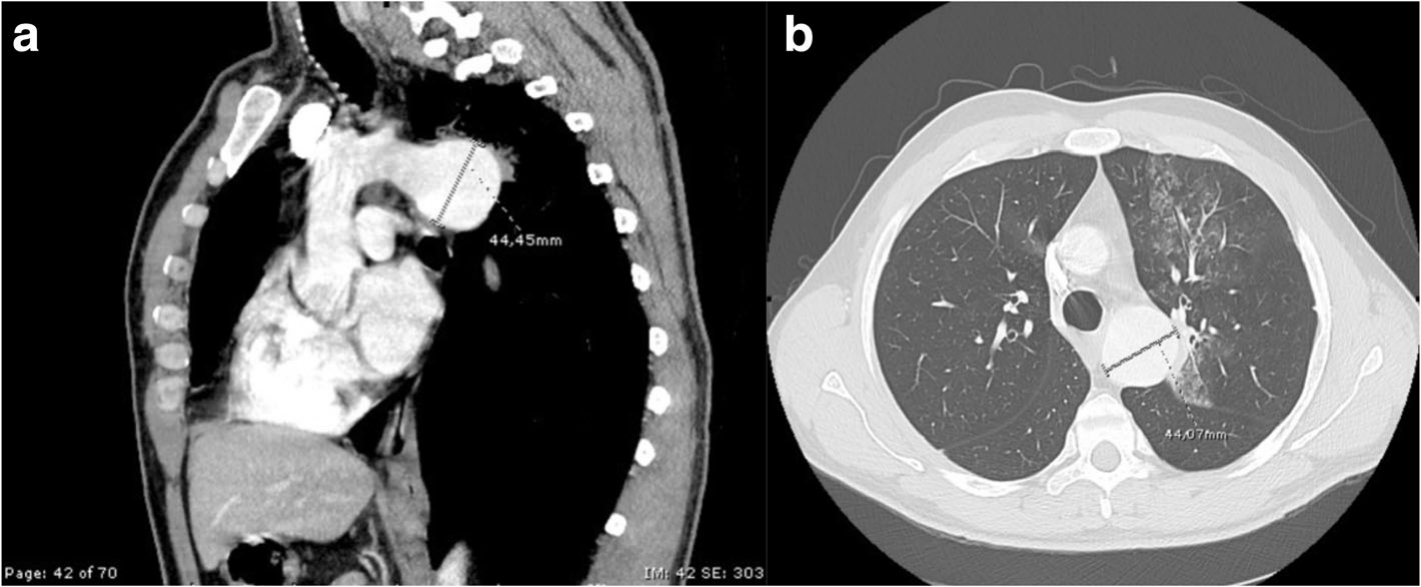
Panel a Longitudinal reconstruction of chest CT scan image showing the aneurysmal dilatation of thoracic aorta. Panel b Axial reconstruction of chest CT images showing the maximal diameter of the aortic aneurism (44,07 mm) and the ground glass opacities at the left upper lobe
